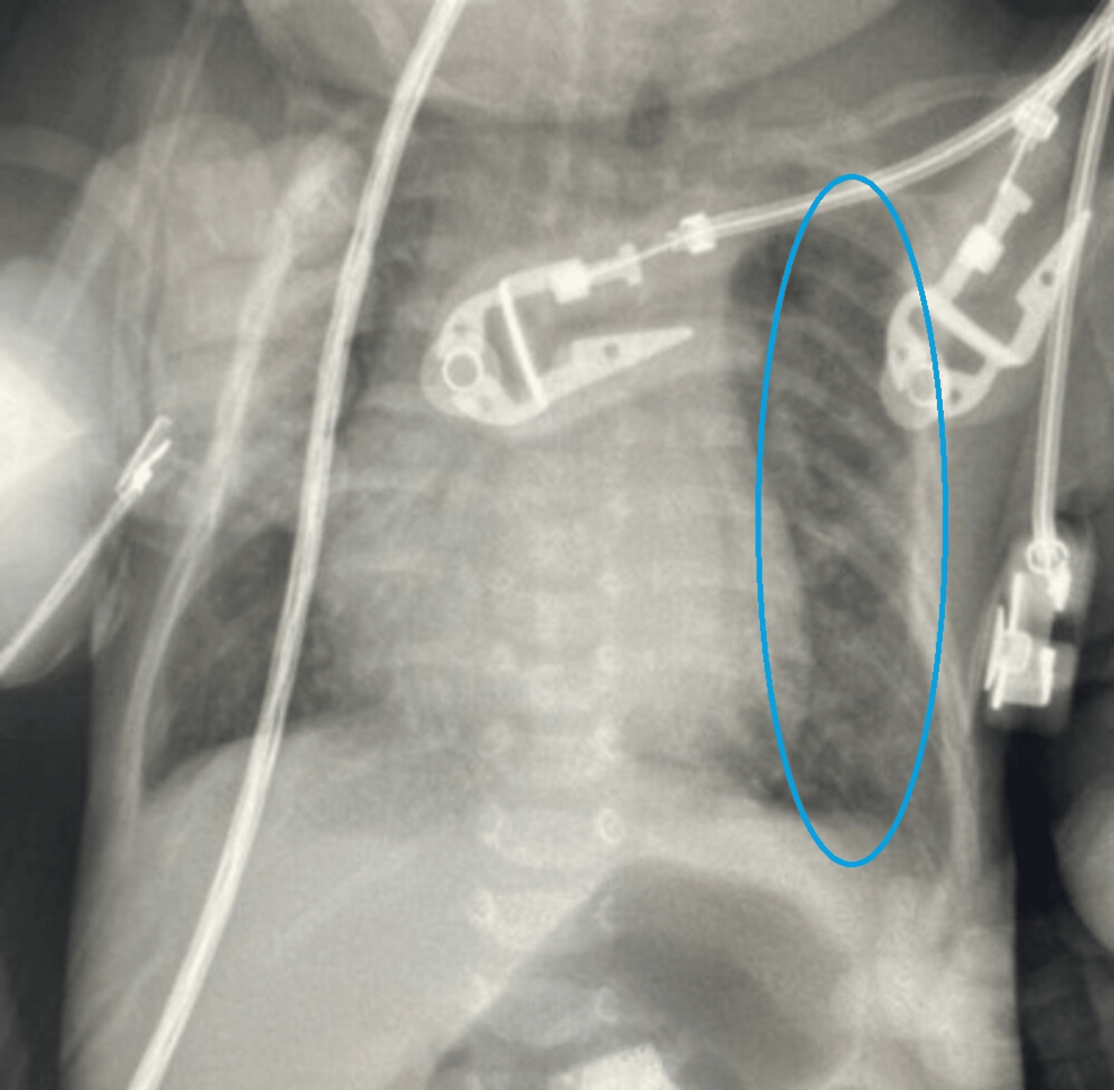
Postoperative radiograph The image shows absence of the mass (red circle) and return of the mediastinal structures to the midline position.
